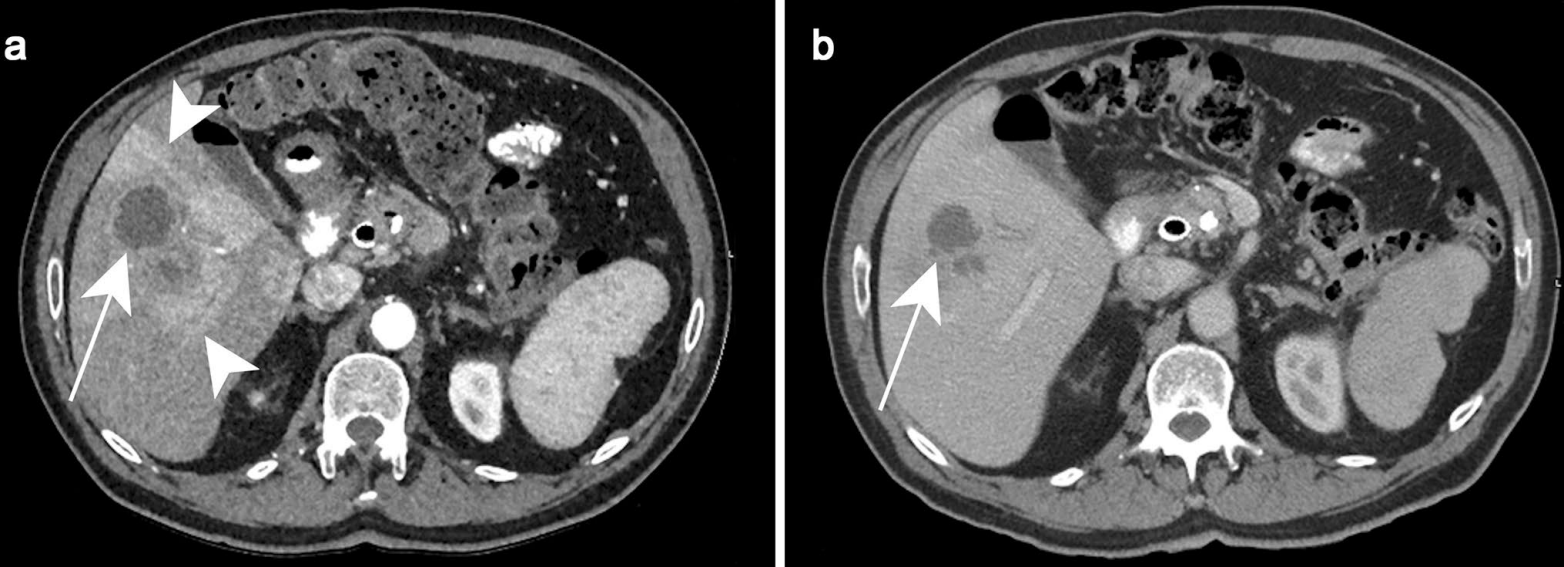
Pyogenic abscess in a 60-year-old female patient with a history of chronic pancreatitis who presented with asthenia and fever. Axial arterial phase (a) and portal venous phase (b) contrast-enhanced CT show small clustering lesions with a dominant hypoattenuating lesion in segment V of the liver (white arrows) corresponding to pyogenic hepatic abscesses. Altered perfusion disorder is observed as geographic areas of hyperattenuation peripheral to the hepatic abscesses (white arrowheads), clearly visualized on arterial phase (a)
